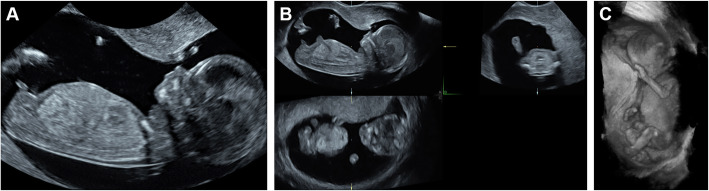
Different imaging techniques of the same pregnancy in the first trimester. Images, representing respectively transvaginal two-dimensional (panel a), three-dimensional (panel b) and three-dimensional virtual reality (Panel c) ultrasound images of the same pregnancy in the first trimester
